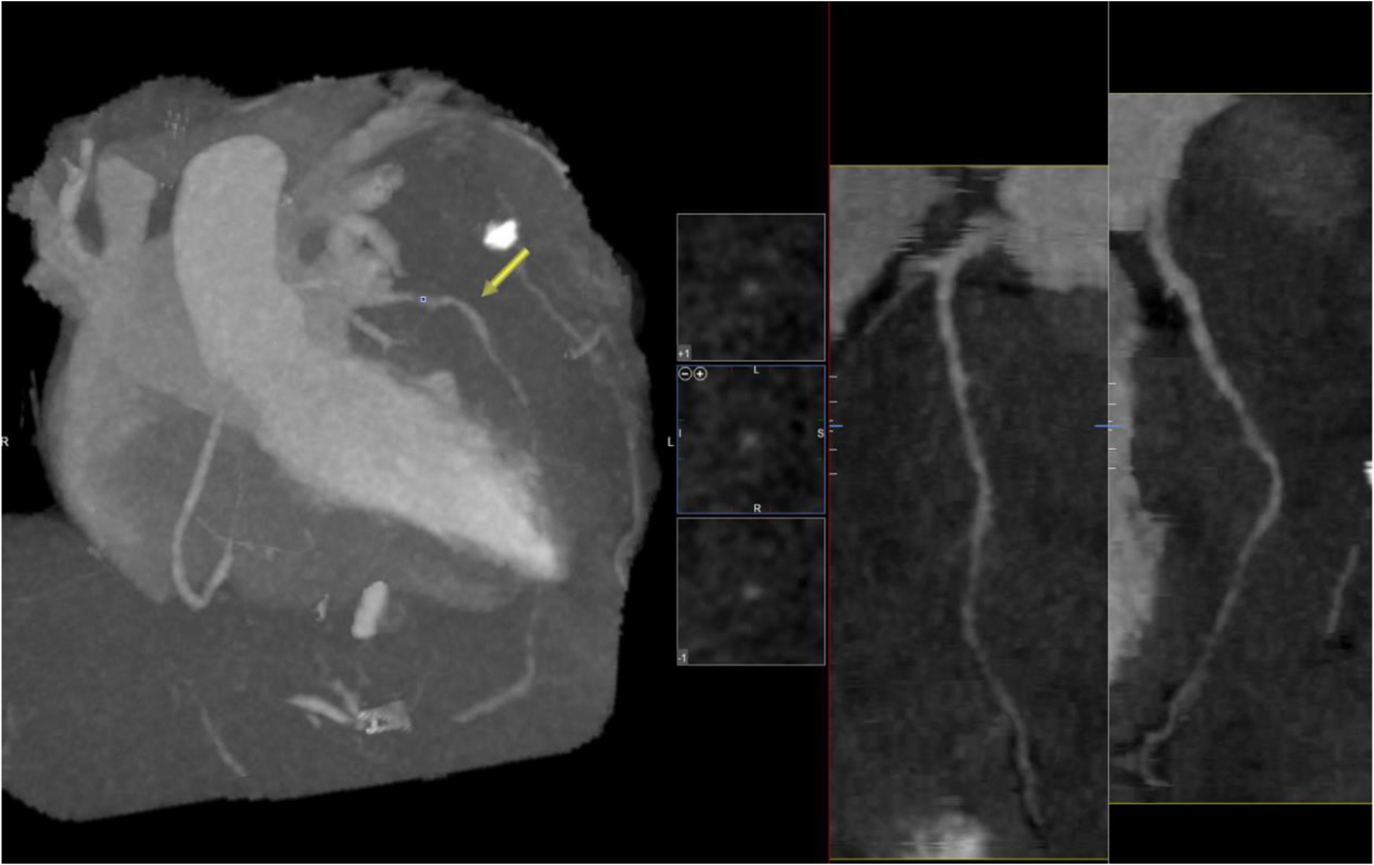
CT coronary angiogram reveals displacement of the left anterior descending coronary artery (LAD) yellow arrow. Right anterior oblique projection
